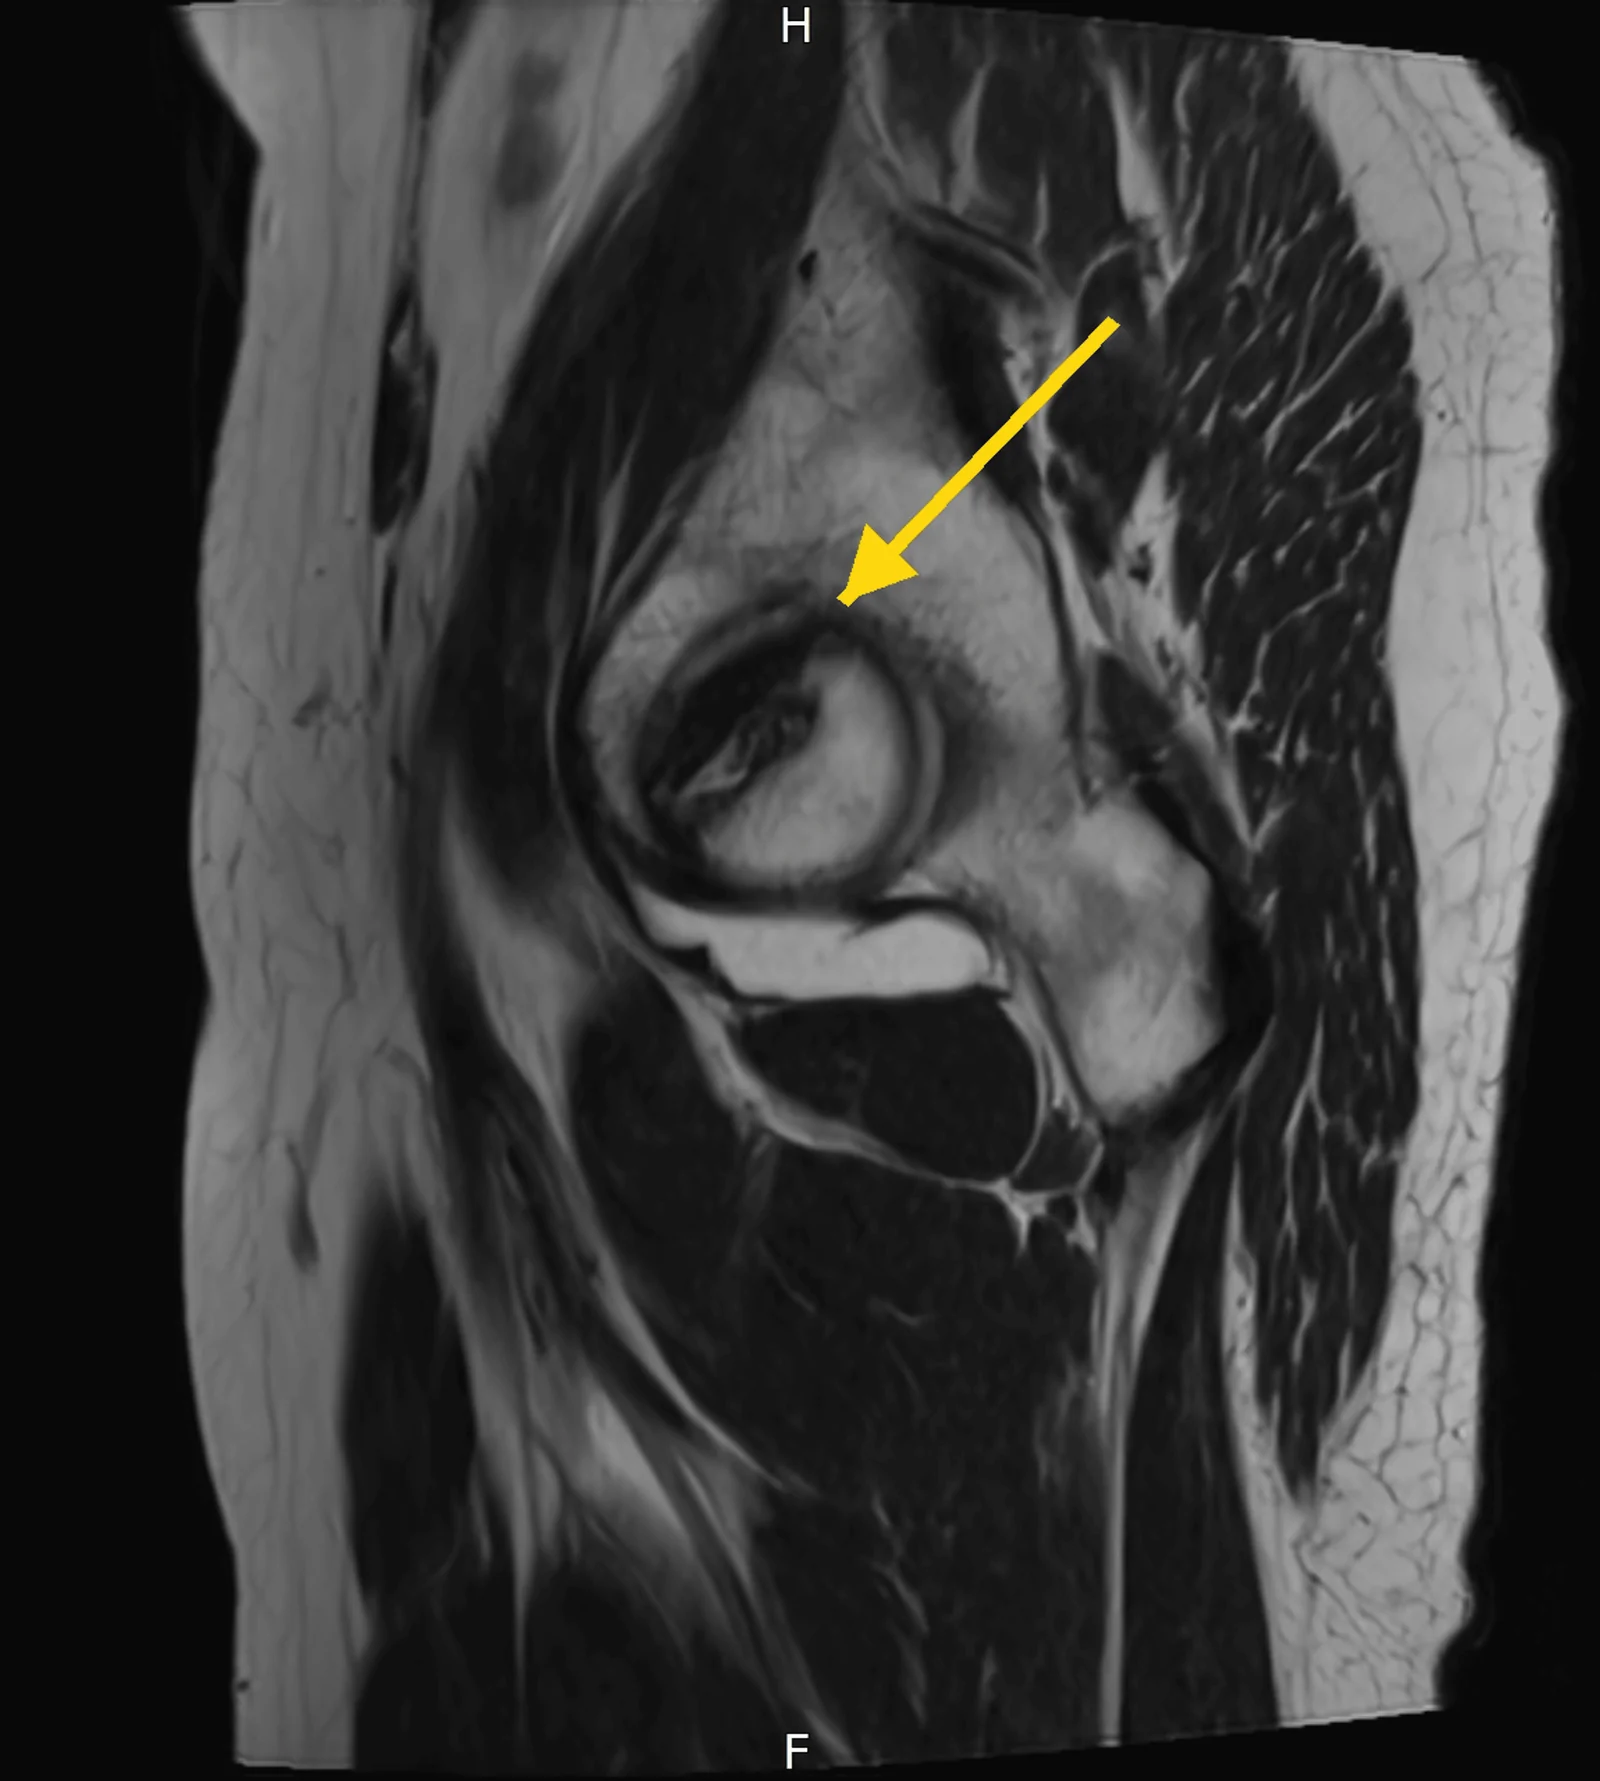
Sagittal MR image of the right hip The arrow indicates the femoral head lesion without subchondral collapse, consistent with Ficat-Arlet stage II disease.
